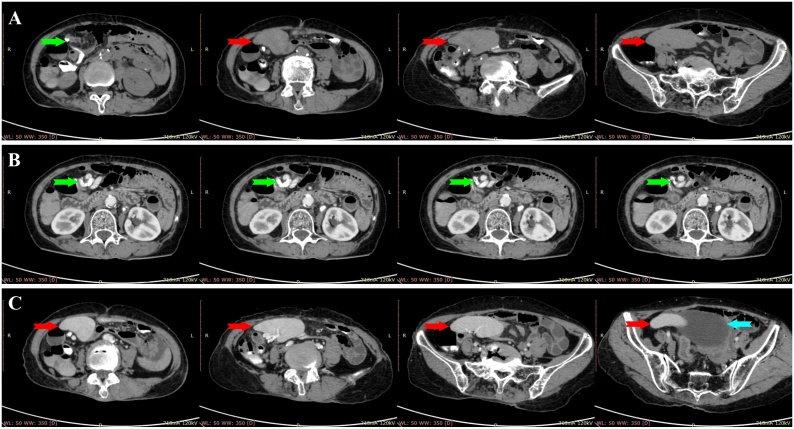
(A): Axial view of the abdominal non-contrasted CT scan showing a suspicious pedicle (green arrow) and an ectopic spleen (red arrow) in the right abdominal and pelvic cavities. (B): Axial view of the abdominal contrasted CT scan showing a twisted splenic vascular pedicle (green arrow) in a whirlpool disposition. (C): Axial view of the abdominal contrasted CT scan showing an enlarged ectopic spleen (red arrow) extending from the right abdominal cavity to the pelvic cavity, lateral to the urinary bladder (blue arrow).
